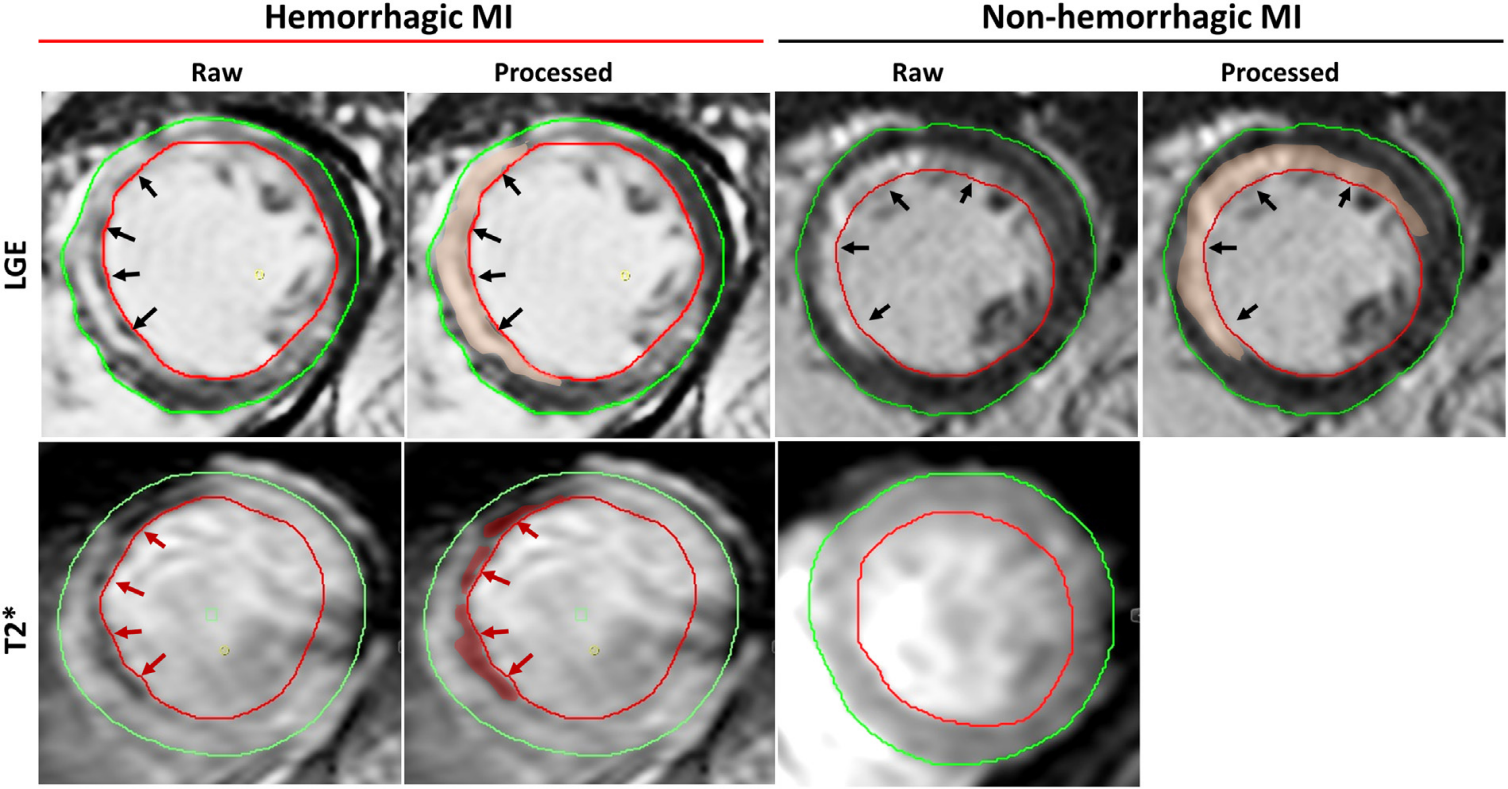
Representative cardiovascular magnetic resonance (CMR) images of intramyocardial hemorrhage (IMH). LGE, late gadolinium enhancement; MI, myocardial infarction; T2∗, T2-weighted images with multiple echo times.
